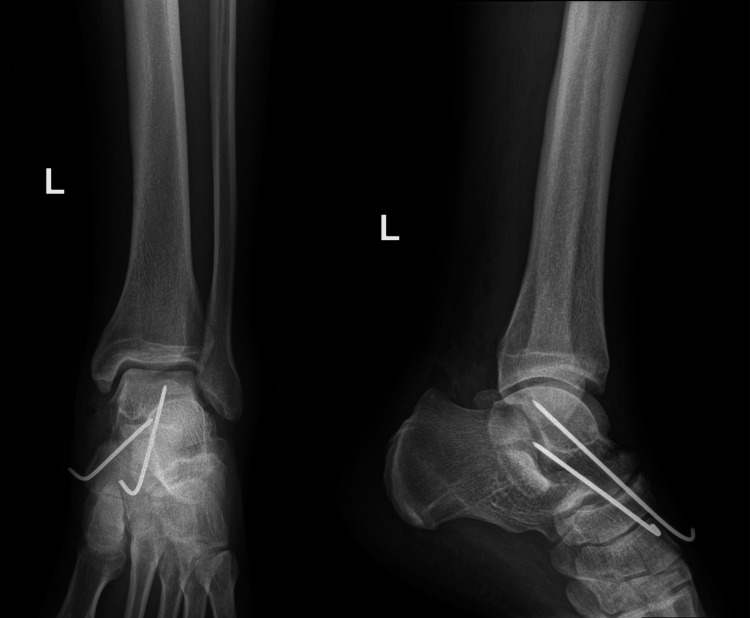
Anteroposterior (AP) and lateral view X-rays of the left ankle after surgery
